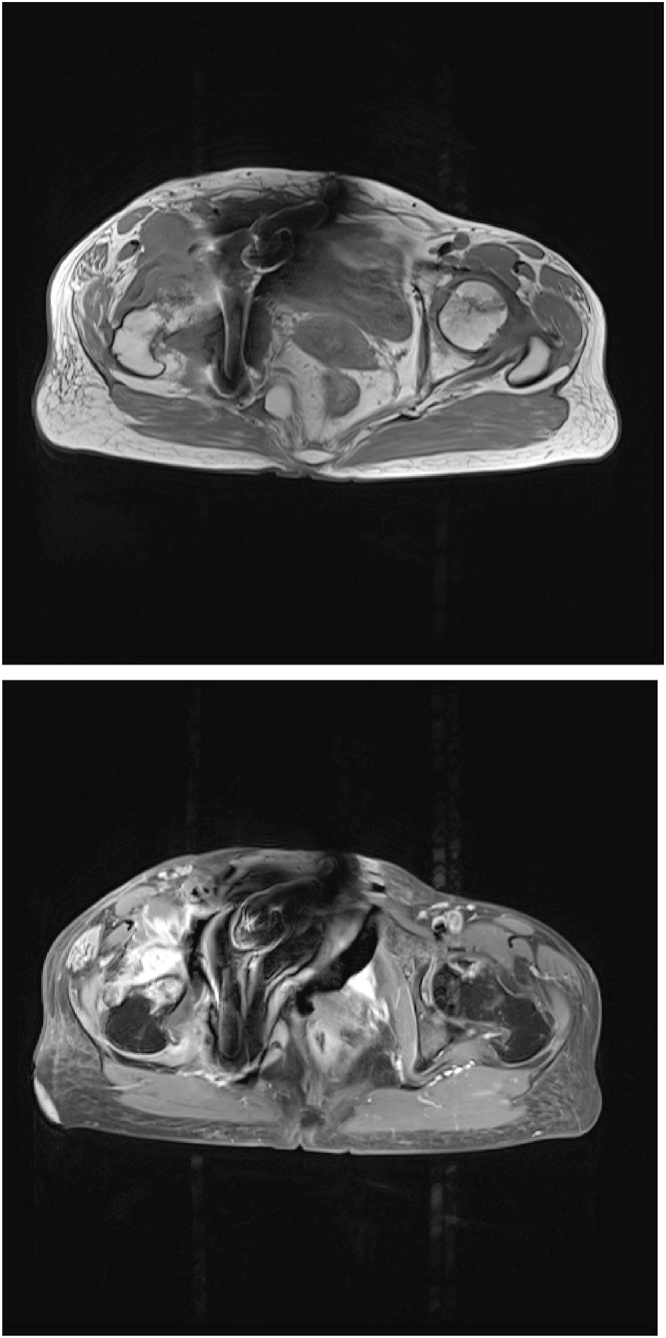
MRI T1 images showing bone marrow oedema of the femoral head with articular depression at the weight bearing surface with synovitis, synovial hypertrophy and large effusion.
